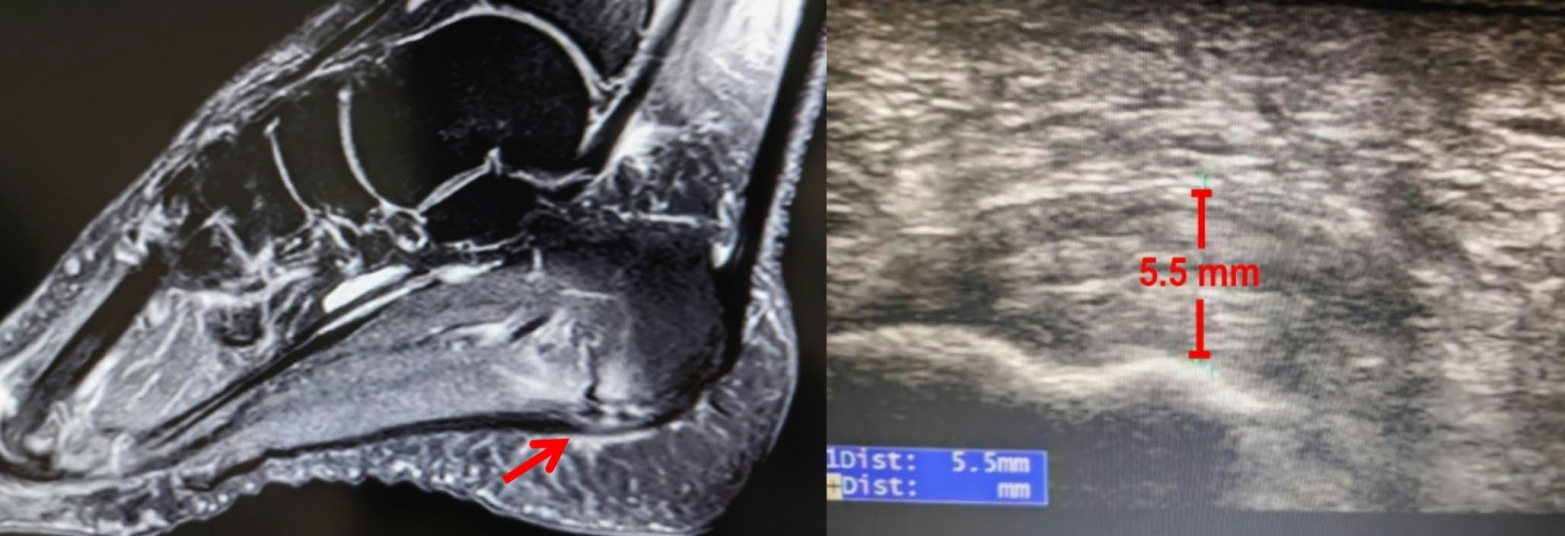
Preoperative MRI and ultrasound revealed an inflamed and thickened plantar fascia (red arrow). It indicative of chronic stress and inflammation. The MRI provided detailed visualization of soft tissue structures, confirming the extent of inflammation and any associated abnormalities. Ultrasound, as a dynamic and accessible tool, further highlighted the localized thickening and areas of increased vascularity, characteristic of plantar fasciitis
